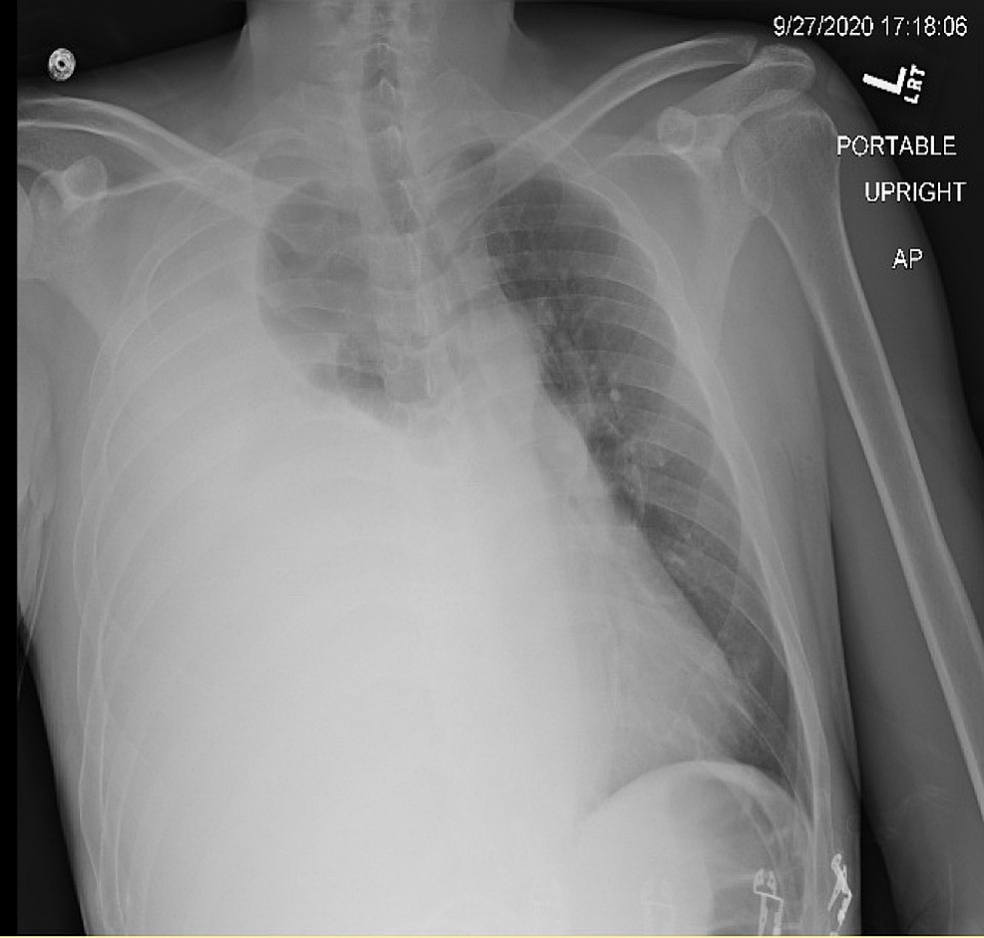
Chest X-ray upon admission Chest X-ray showing opacification of the right hemithorax and a deviated trachea
